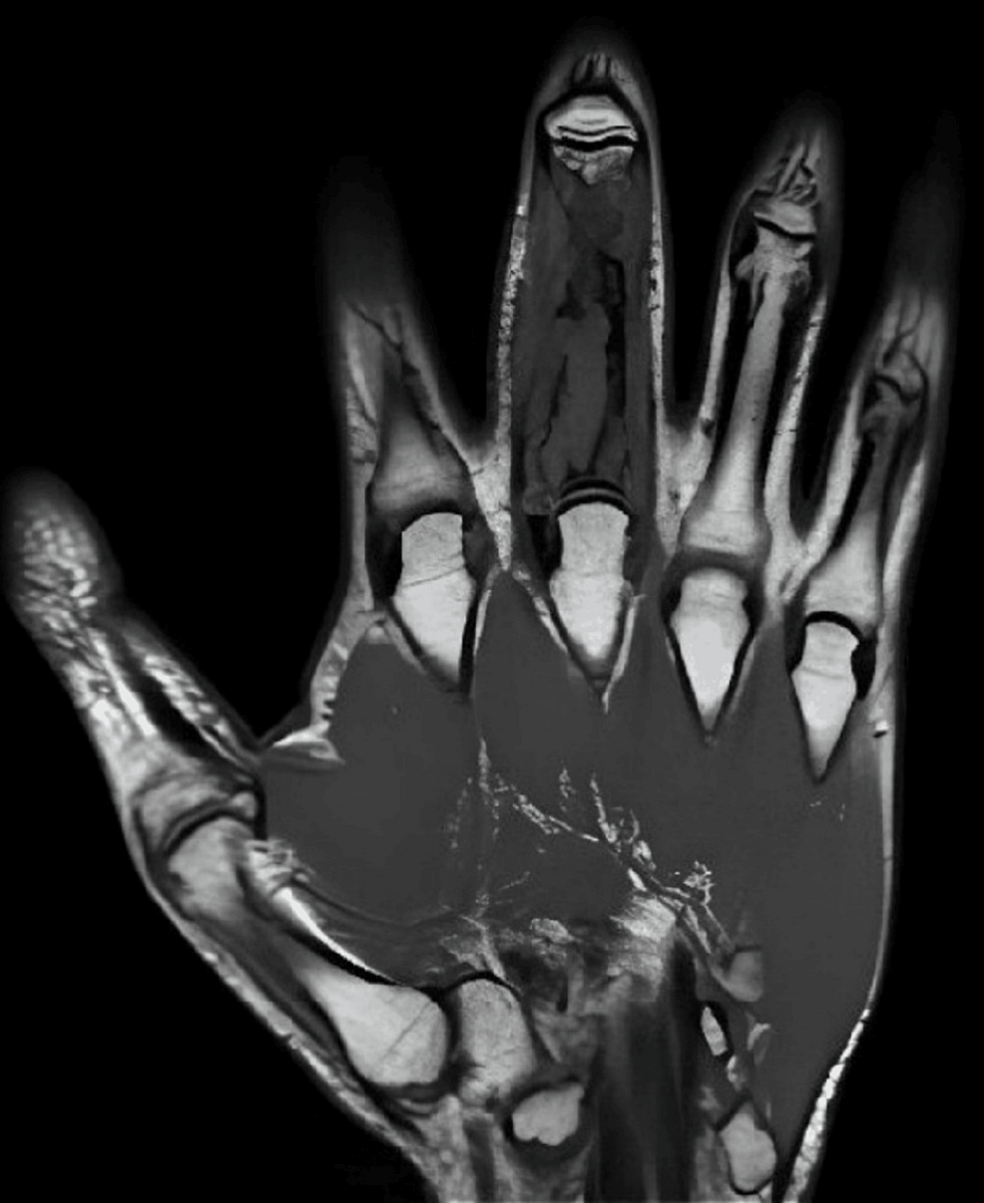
Coronal T1-weighted magnetic resonance imaging of the right hand after neoadjuvant treatment
